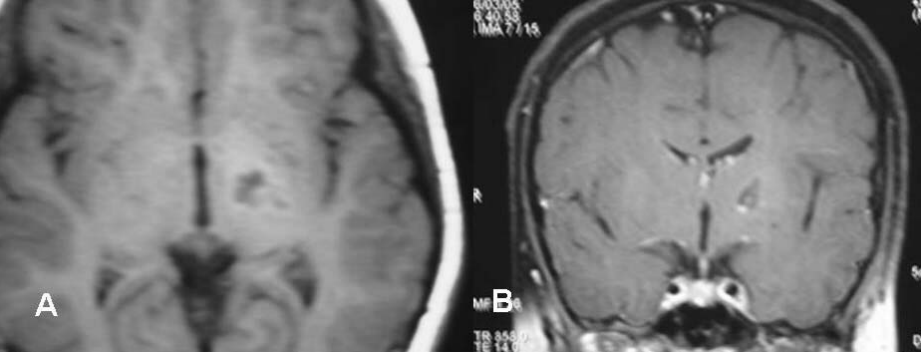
MRI brain follow-up after 30 months of Gamma Knife surgery of left thalamic recurrent pilocytic astrocytoma with marked reduction of the lesion size.
